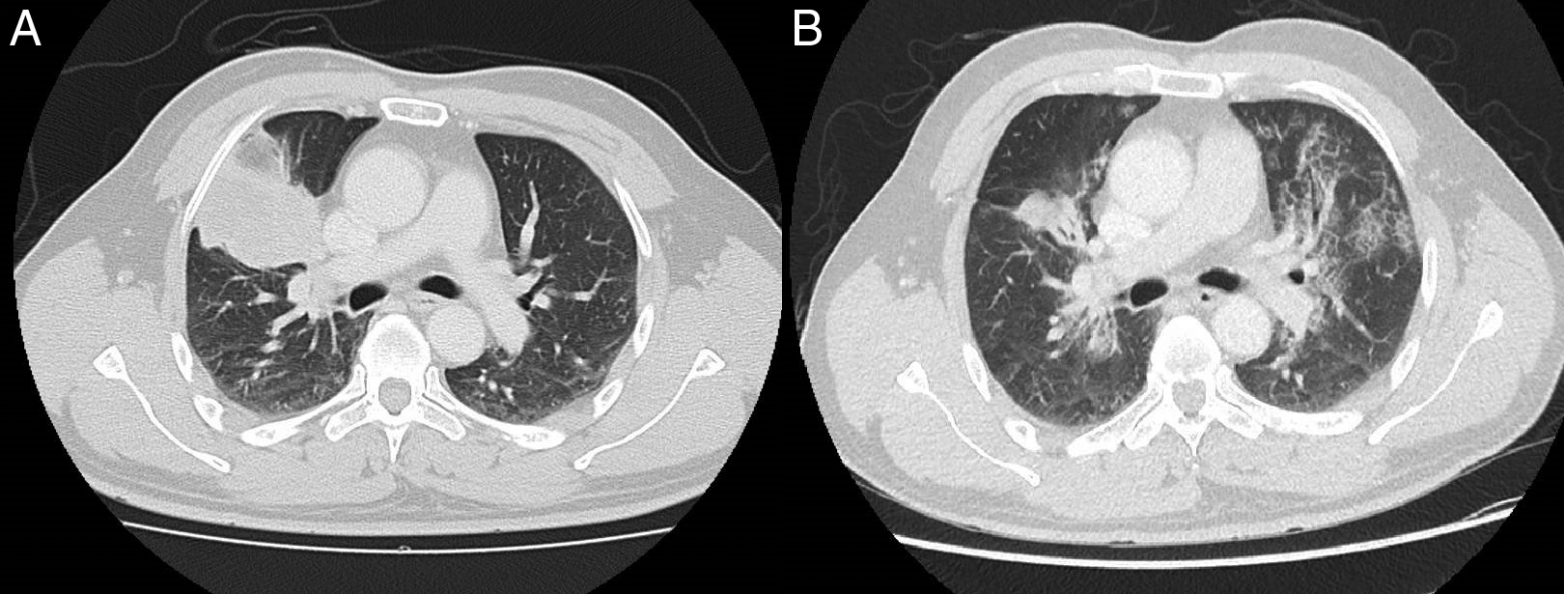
(A) Computed tomography (CT) thorax with right upper lobe pulmonary cryptococcoma at initial presentation. (B) CT thorax 15 months after initial of anti‐fungal therapy, showing decreased size of right upper lobe pulmonary cryptococcoma and development of new crazy‐paving pattern.
